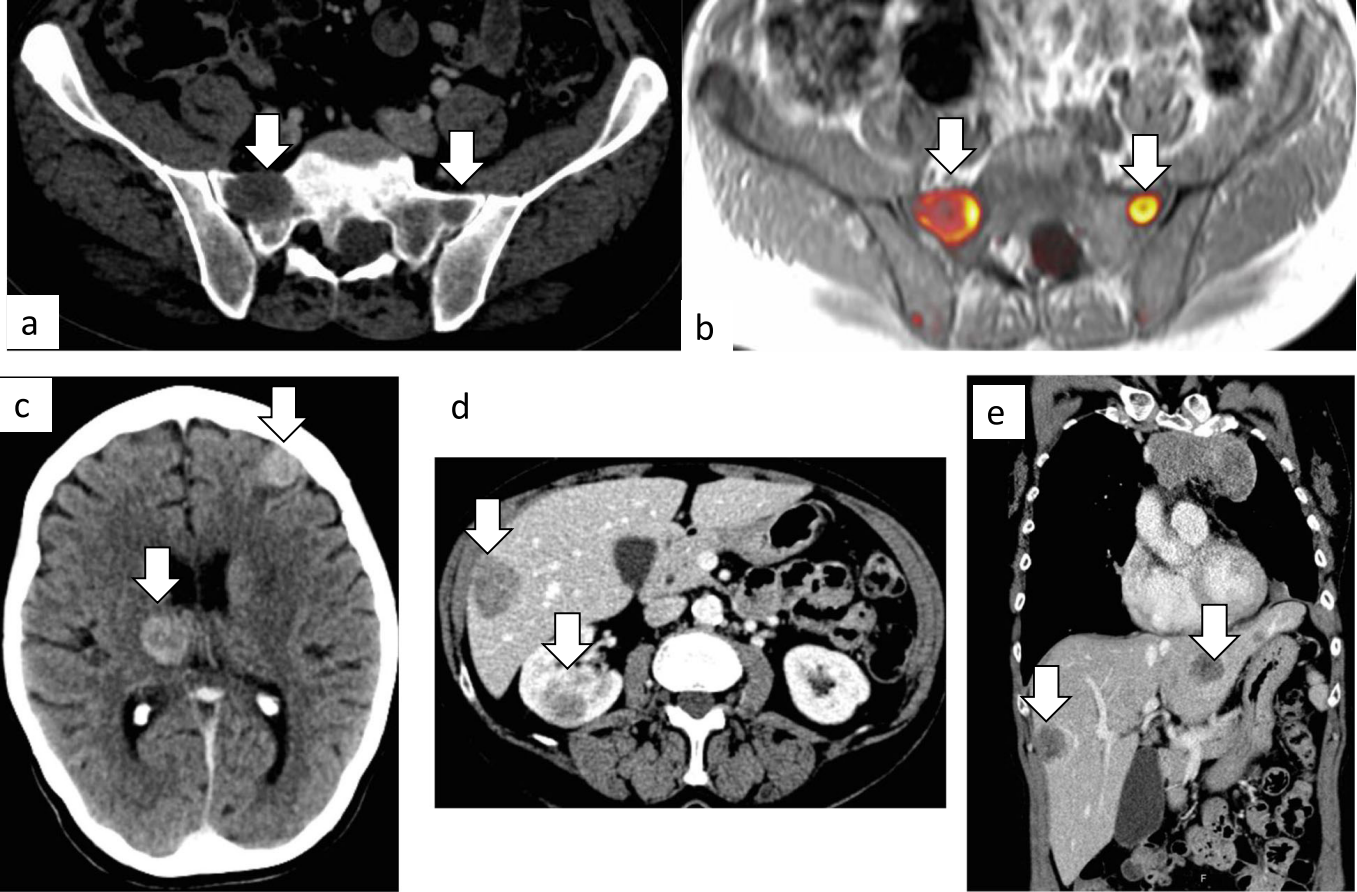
Radiological imaging of distant metastatic sites. Axial contrast enhanced CT (a) and fused DWI-T1W-MRI (b) images showing lytic bone metastases in the sacrum bilaterally. Axial contrast enhanced CT showing brain metastases (c). Axial enhanced CT (d) showing liver and renal metastases. Coronal enhanced CT (e) showing liver metastases. *CT: computer tomography; DW1: diffusion weighted 1, T1W: T1 weighted, MRI: magnetic resonance imaging*
